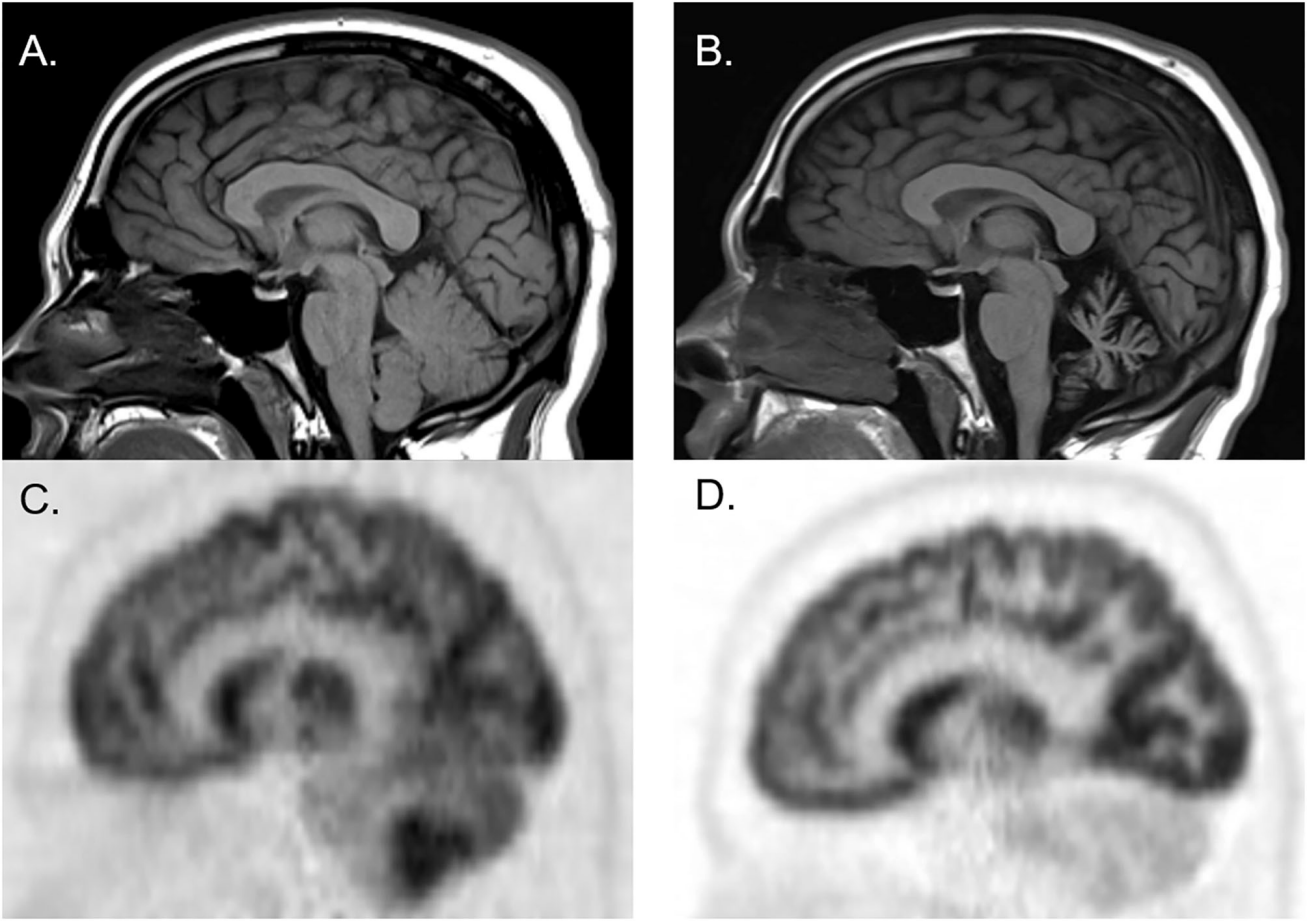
Brain MRI and FDG-PET CT of a man who initially presented with cerebellar ataxia with elevated serum anti-glutamic acid decarboxylase-65 antibodies. At initial presentation, brain MRI was normal [(A) sagittal], whereas brain FDG-PET showed hypermetabolism of cerebellar hemispheres [(C) sagittal]. Three years later, follow-up brain MRI showed marked cerebellar atrophy [(B) sagittal], and brain FDG-PET showed hypometabolism of cerebellar hemispheres [(D) sagittal].
